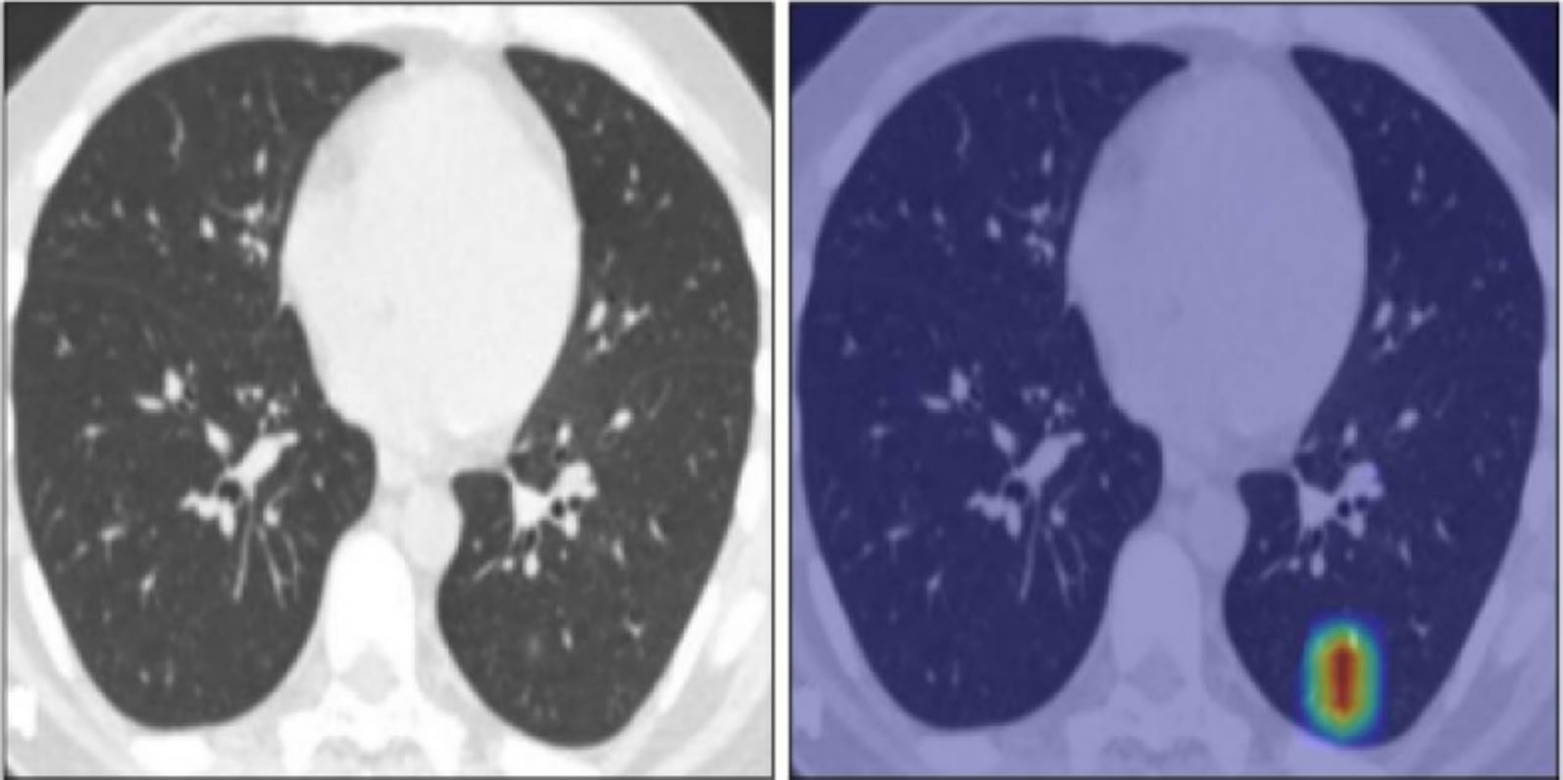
A 36-year-old male patient presented with cough, chest pain and bone ache, yet CT chest was unremarkable and after doing color-coded images an ill-defined left lower lobar faint small ground glass nodule became more evident
